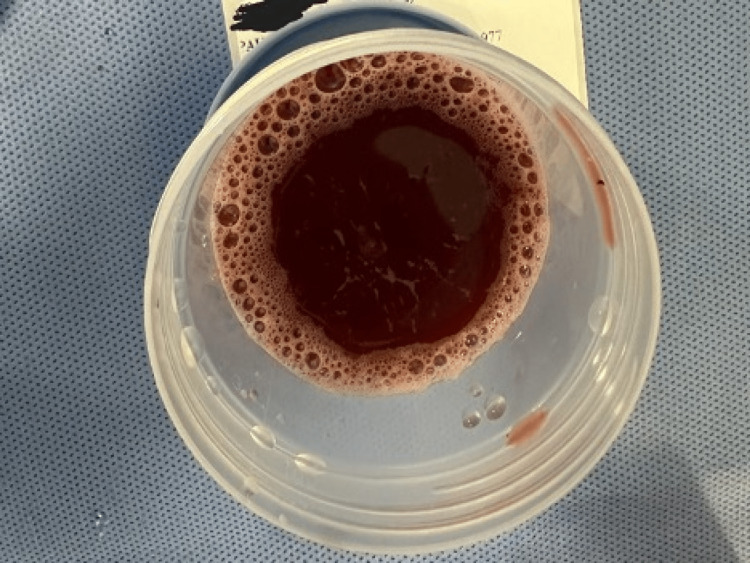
Grossly hemorrhagic fluid obtained with CT guidance. CT: computed tomography
